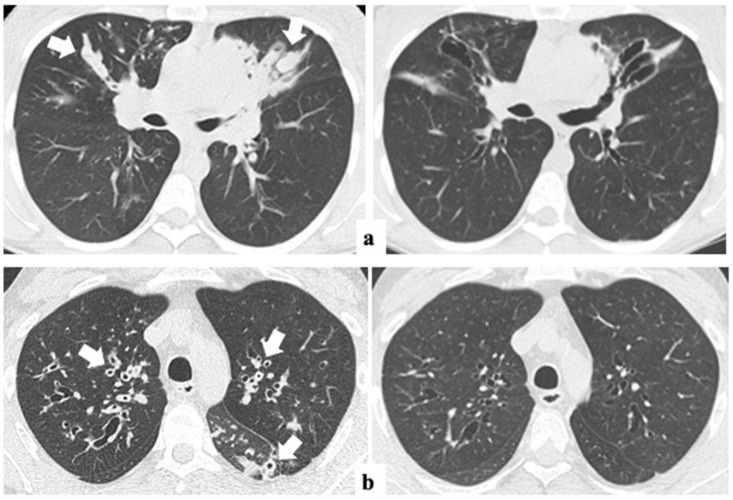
Axial lung CT images before (left) and after one-year ETI therapy (right) in two adults with CF. Dramatic improvements in mucus plugs in (a) (arrows) and peribronchial thickening in (b) (arrows) are shown. ETI: elexacaftor–tezacaftor–ivacaftor.
